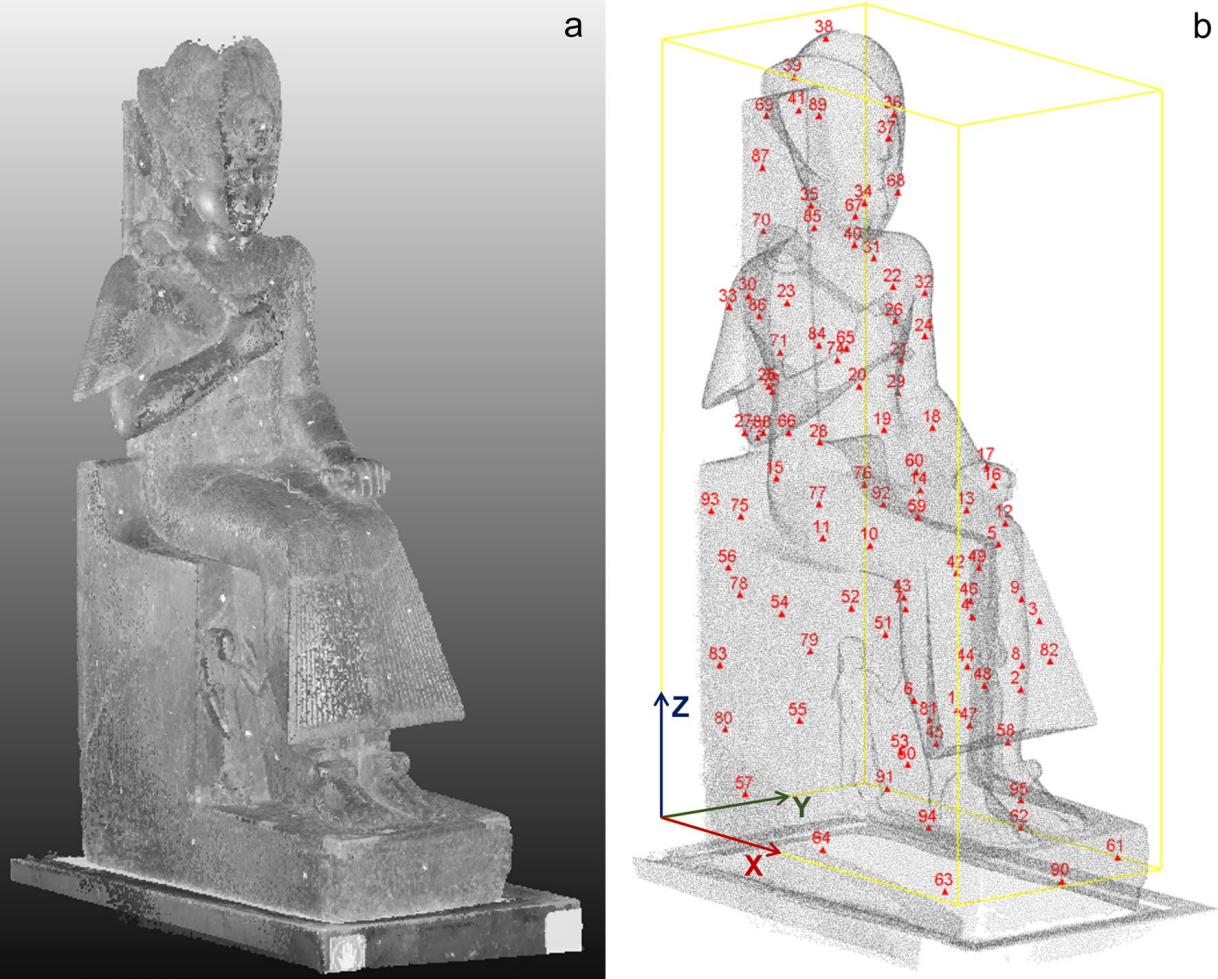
3D model of the statue of Ramses II. (a) Point cloud with RGB colors obtained with ground-based laser scanning. (b) Complete point cloud obtained through handheld and ground-based laser scanning, with indication of the 3D ultrasonic tomography source and receiver locations (red triangles, georeferenced through total station measurements) and of the local reference system adopted in the study. Figure created in CloudCompare (version 2.12.2, https://www.danielgm.net/cc/).
